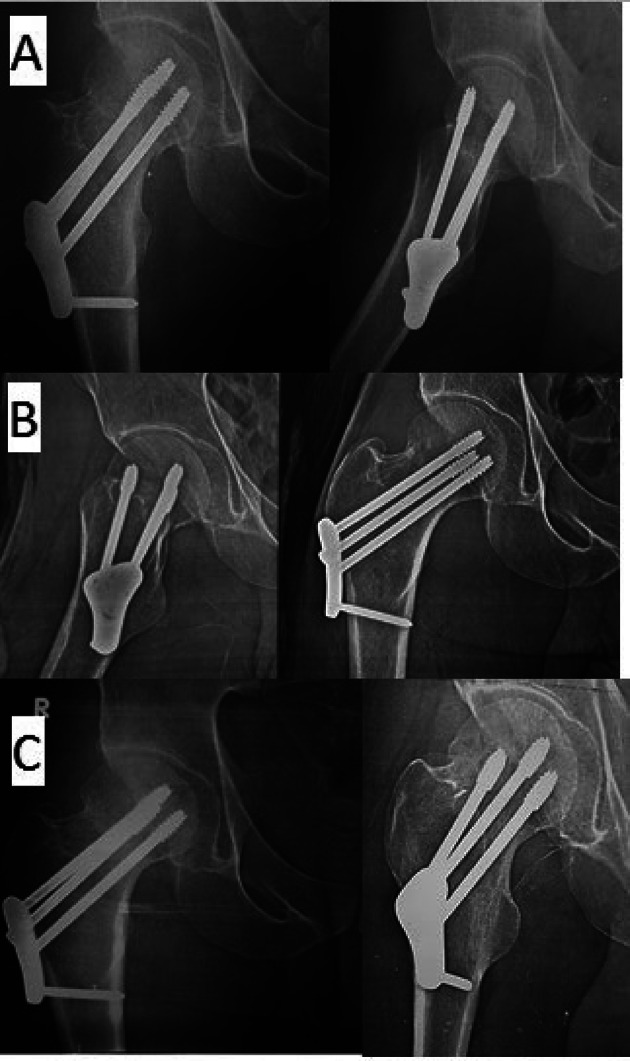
Follow up radiographs of the patient treated by FNLP. A X-rays at 2nd month post-operatively showing partial fracture healing. B Complete fracture healing and stable internal fixation at the 4th month postoperatively. C X-rays at 12th month follow up
